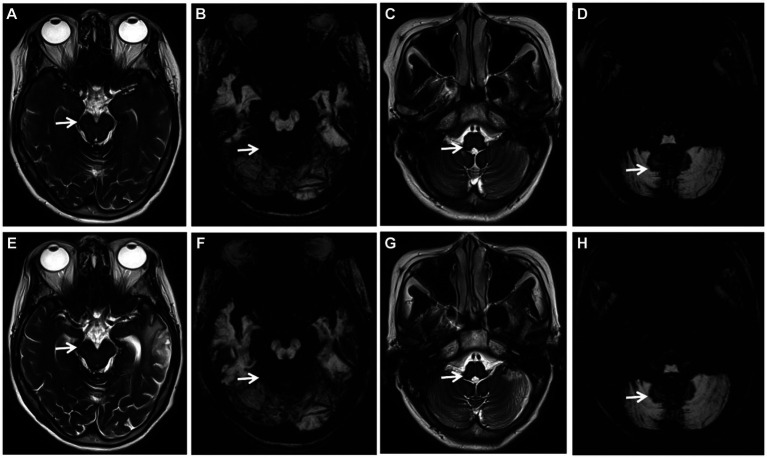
(Case 1) Axial T2-weighted images (WI) (A, C, E, G; arrows) and axial susceptibility-weighted imaging (SWI) (B, D, F, H; arrows) showing extensive SS, no progression of SS before treatment (A–D) and 2 years after deferiprone treatment (E–H).
